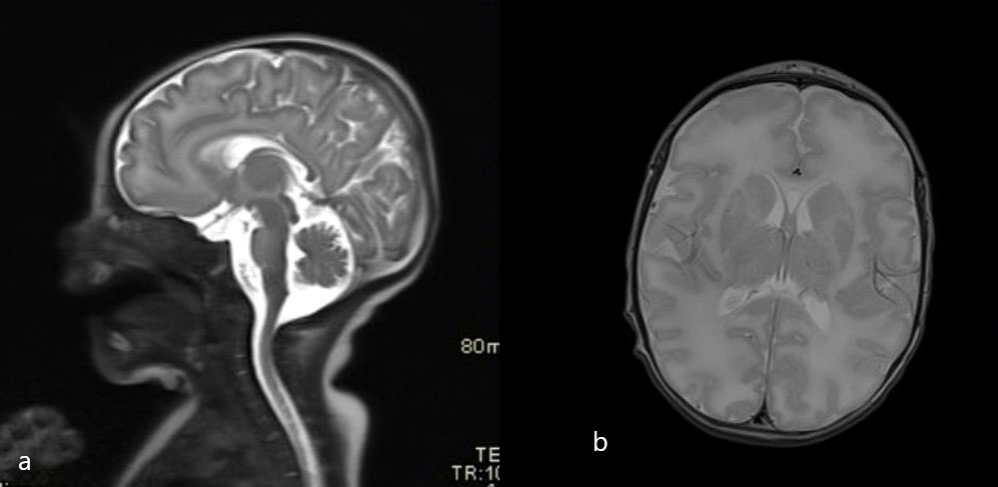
Initial brain MRI scans (T2 sagittal and axial plane). (A) Normal thickness of pons and midbrain. (A, B) Higher T2 signal of the frontal white matter.
